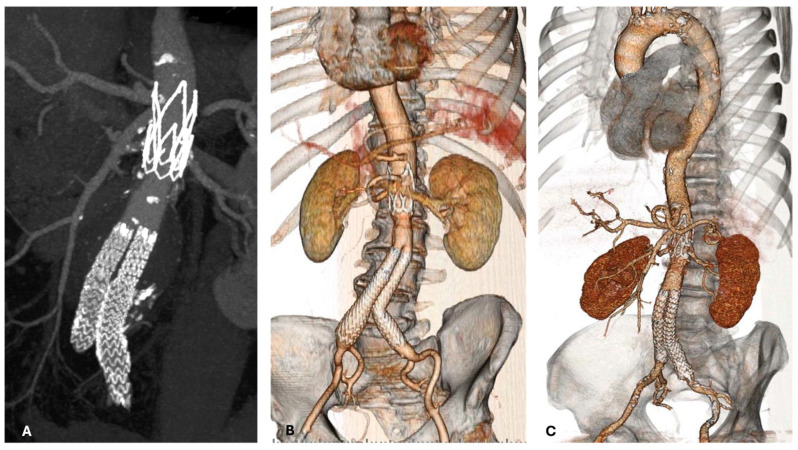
Postoperative CT angiogram demonstrating effective Ovation implantation and aneurysm exclusion in short neck (A,B) and in case of calcified iliac accesses (C).
